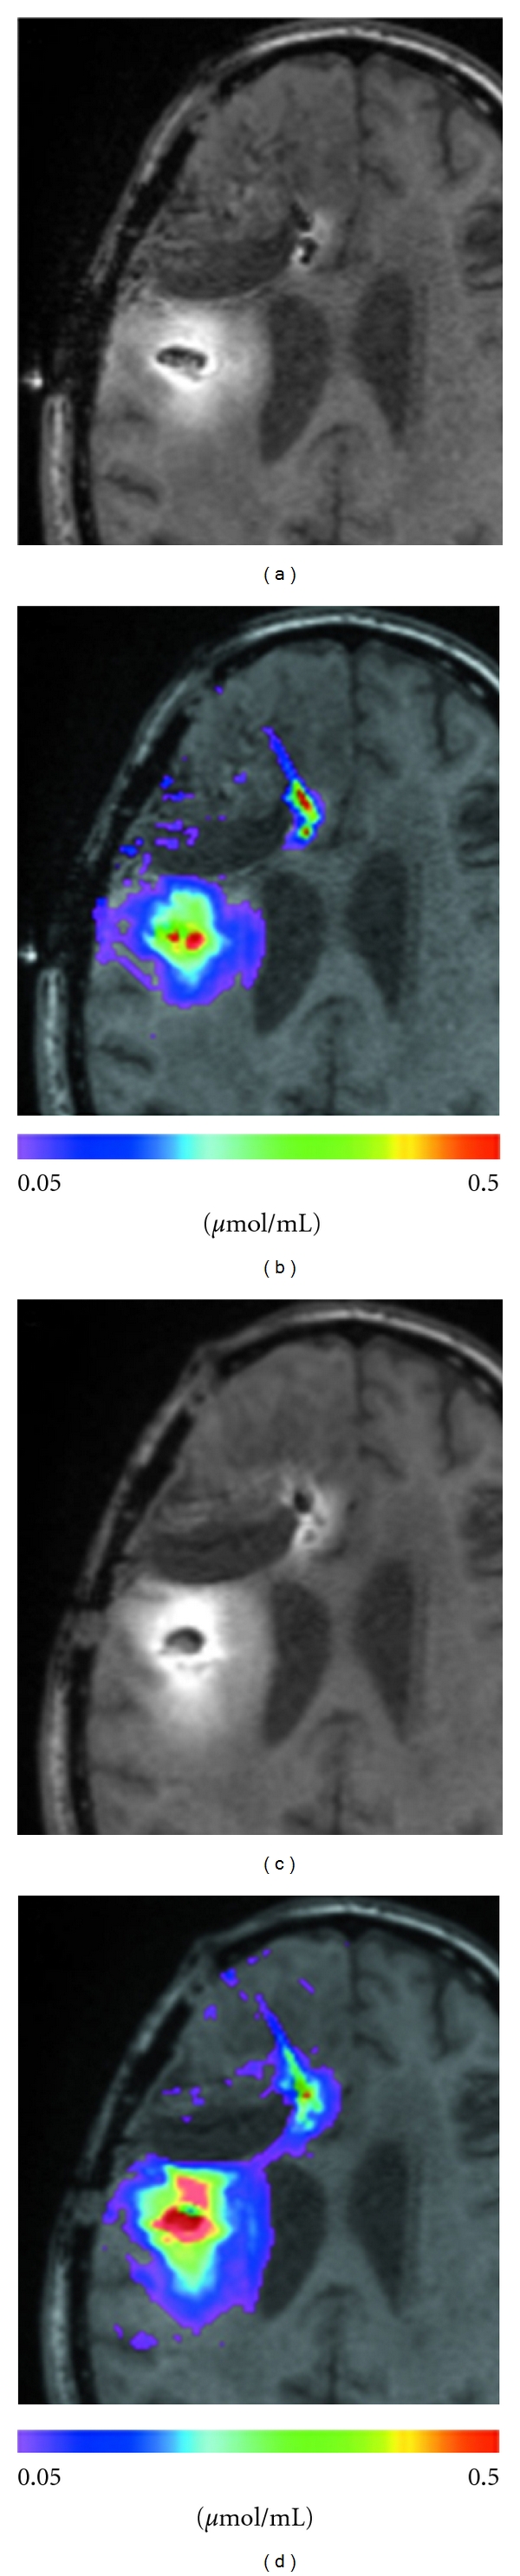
T1-weighted signal of MR1-1-PE38KDEL (a and c), compared with measured concentration profile of Gd-DTPA (b and d). (a) T1-weighted signal of MR1-1-PE38KDEL at 24 hours. (b) Concentration of Gd-DTPA at 24 hours. (c) T1-weighted signal of MR1-1-PE38KDEL at 72 hours. (d) Concentration of Gd-DTPA at 72 hours. Gd-DTPA, gadolinium-diethylene triamine pentaacetic acid. Reprinted from [122] with permission from Wolters Kluwer Health (license number 2747070143830).
